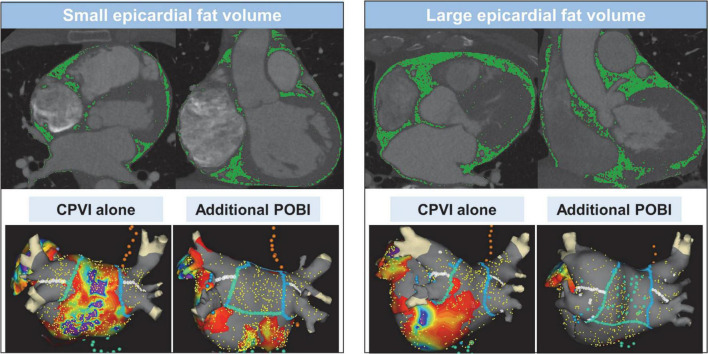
Measurement of epicardial adipose tissue volume using 3-dimensional computed tomography and ablation lesions and voltage map of the strategies of circumferential pulmonary vein isolation alone and additional posterior box isolation in patients with small or large epicardial adipose tissue volume. CPVI, circumferential pulmonary vein isolation; POBI, posterior wall box isolation.
